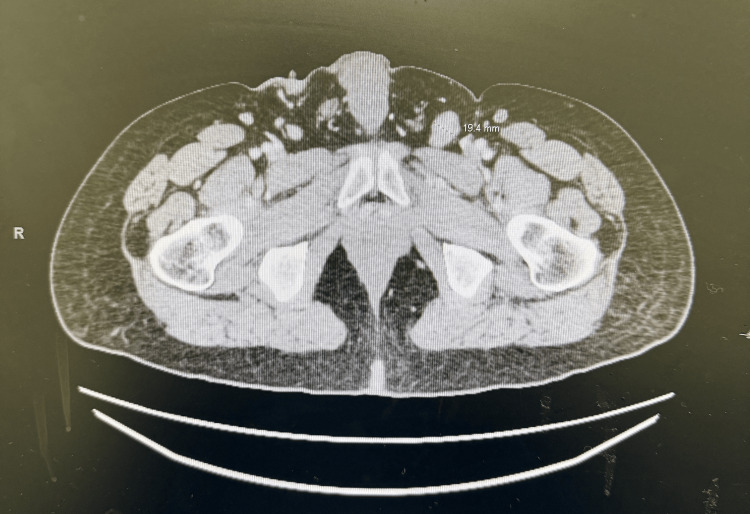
2024 CT pelvis with contrast (multiple axial CT images of the pelvis were obtained with intravenous (IV) contrast to evaluate numerous pelvic masses). Soft tissue thickening and fungating lesions are inseparable from the testicular tissues on this exam. There is a hypodense lesion within the left testicle measuring approximately 1.1 cm. Enlarged left inguinal lymph node measuring up to 1.9 cm and left pelvic sidewall lymph node measuring up to 1.6 cm.
